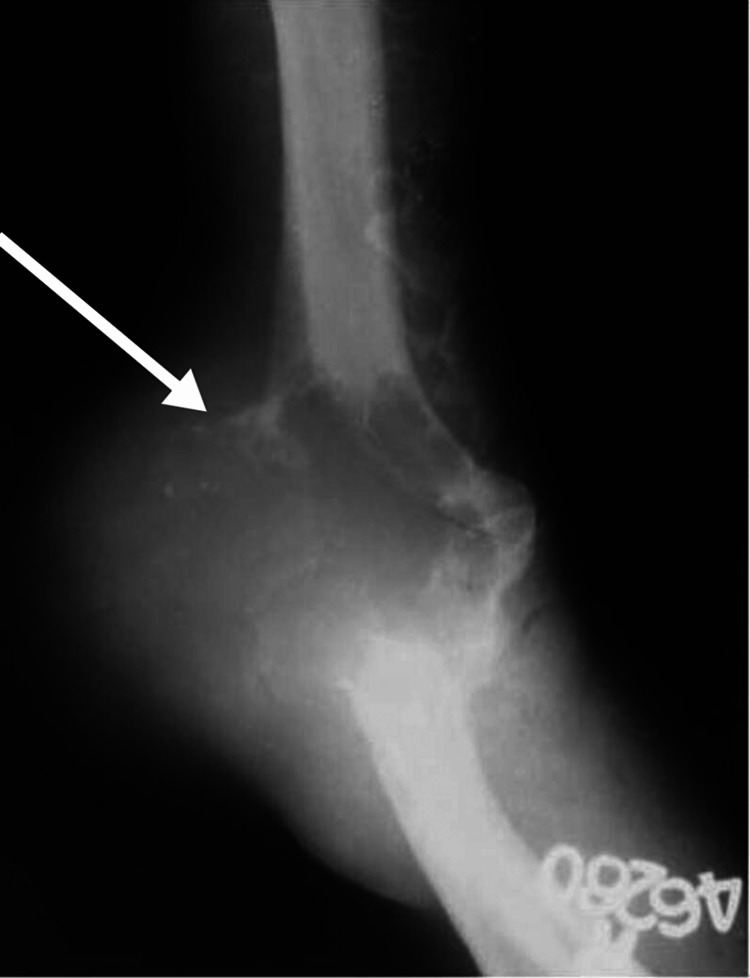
Anteroposterior radiograph of the right elbow after one year. White arrow - Expansile, lytic lesion with cortical breach and soft tissue involvement
